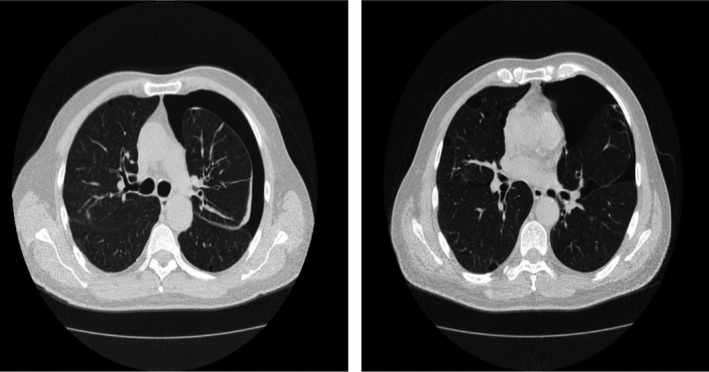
Chest high‐resolution computed tomography of the proband, who experienced recurrent episodes of PSP. Left‐sided PSP and bilateral and multiple bullae are seen as clear. PSP, primary spontaneous pneumothorax
